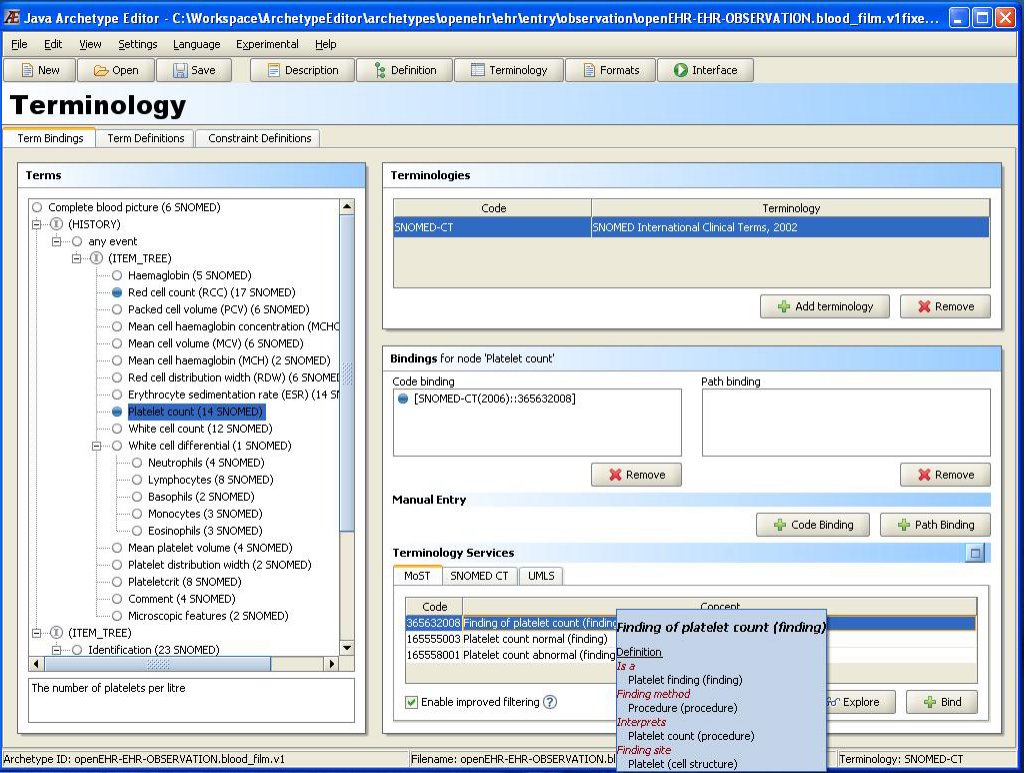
Terminology view. The terminology binding view showing bind suggestions extracted from SNOMED CT by MoST. 14 results were returned by MoST for 'platelet count'. The user has reduced the number of results by selecting only the 'finding' and 'procedure' categories of SNOMED CT. The results can then be related to each other and surrounding context using the built in TermViz feature by clicking the 'Explore' button.
